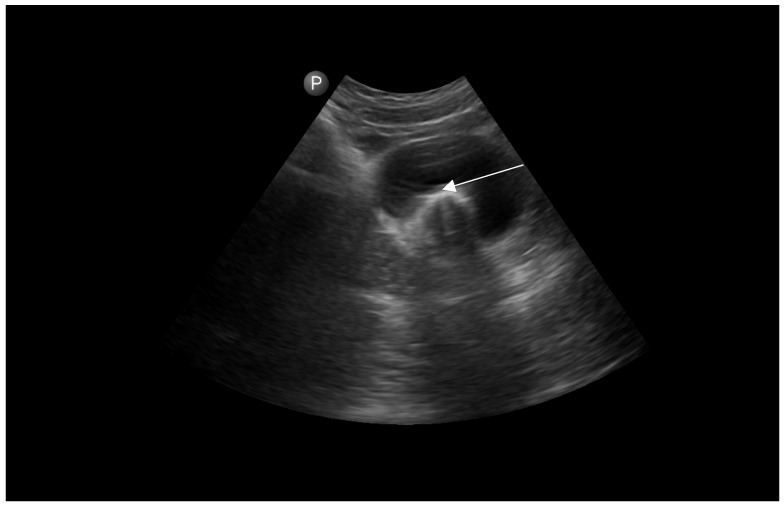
Intraoperative abdominal sonography and puncture of hematocolpos (white arrow). P is polish equivalent to R-right in english site of the ultrasound screen.
